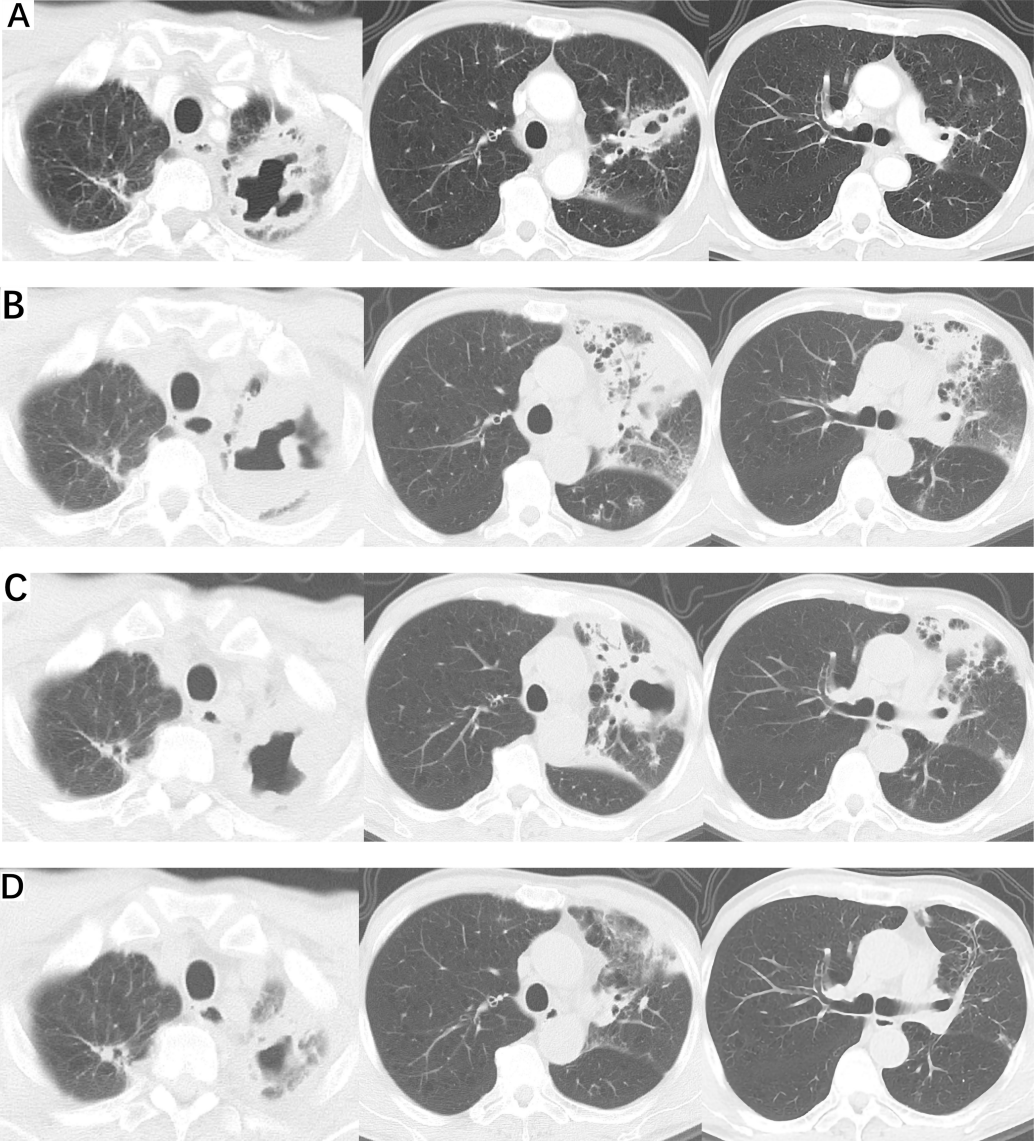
Chest CT on December 18, 2024, demonstrated progression of the left upper lobe cavitary lesion after 6 months of untreated lungs (A). Chest CT on January 3, 2025, showed lesion progression after antibiotic therapy (Levofloxacin first, then linezolid plus cefoperazone sulbactam) (B). Chest CT on January 9, 2025, showed the lesion was not absorbed after antibiotic therapy (linezolid plus cefoperazone sulbactam) (C). Chest follow‐up CT on March 18, 2025, showed significant resolution of pulmonary lesions after two months stopping lenvatinib (D).
